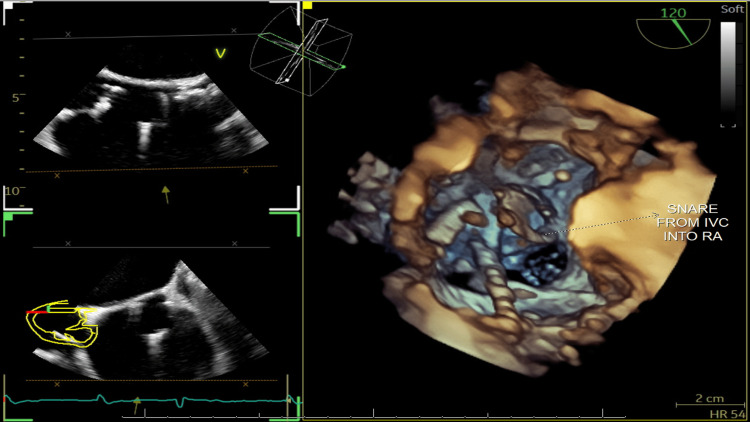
Three-Dimensional Transesophageal Echocardiographic Guidance of Femoral Snare Capture During Retained ICD Lead Extraction Three-dimensional transesophageal echocardiography demonstrates advancement of the femoral snare from the inferior vena cava into the right atrium during retrieval of the retained lead. Real-time spatial imaging facilitated precise snare positioning and successful capture of the lead after multiple conventional retrieval attempts were unsuccessful. ICD: implantable cardioverter-defibrillator
